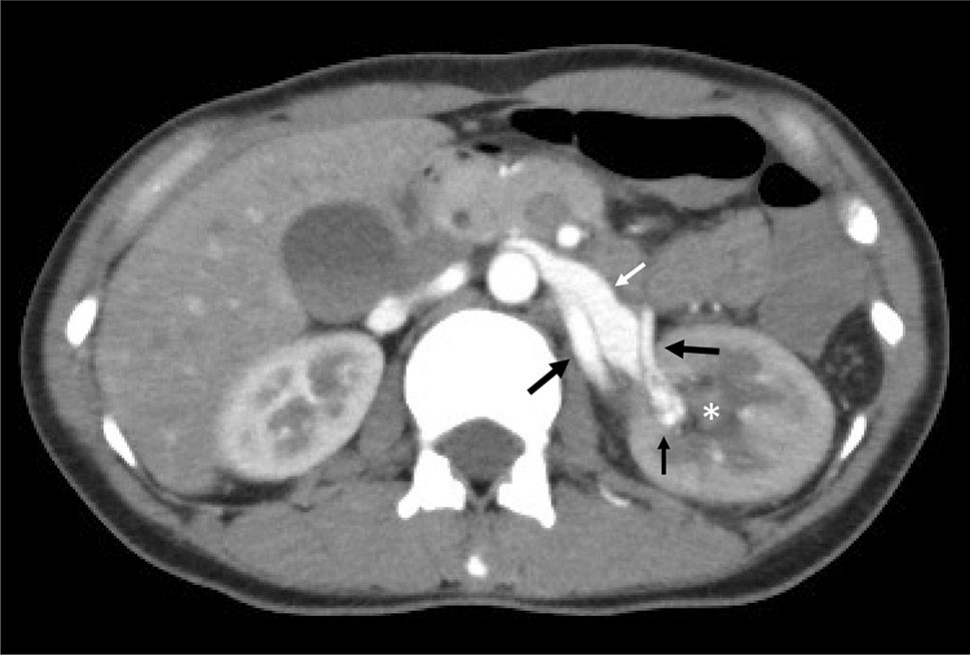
CT reveals urinary tract hematoma and inflow of contrast medium into the left renal vein from a pseudoaneurysm on a branch of the left renal artery in the early arterial phase. *Urinary tract hematoma, white arrow: dilated left renal vein, small black arrow: pseudoaneurysm or AVF, large black arrow: left renal artery and branches
